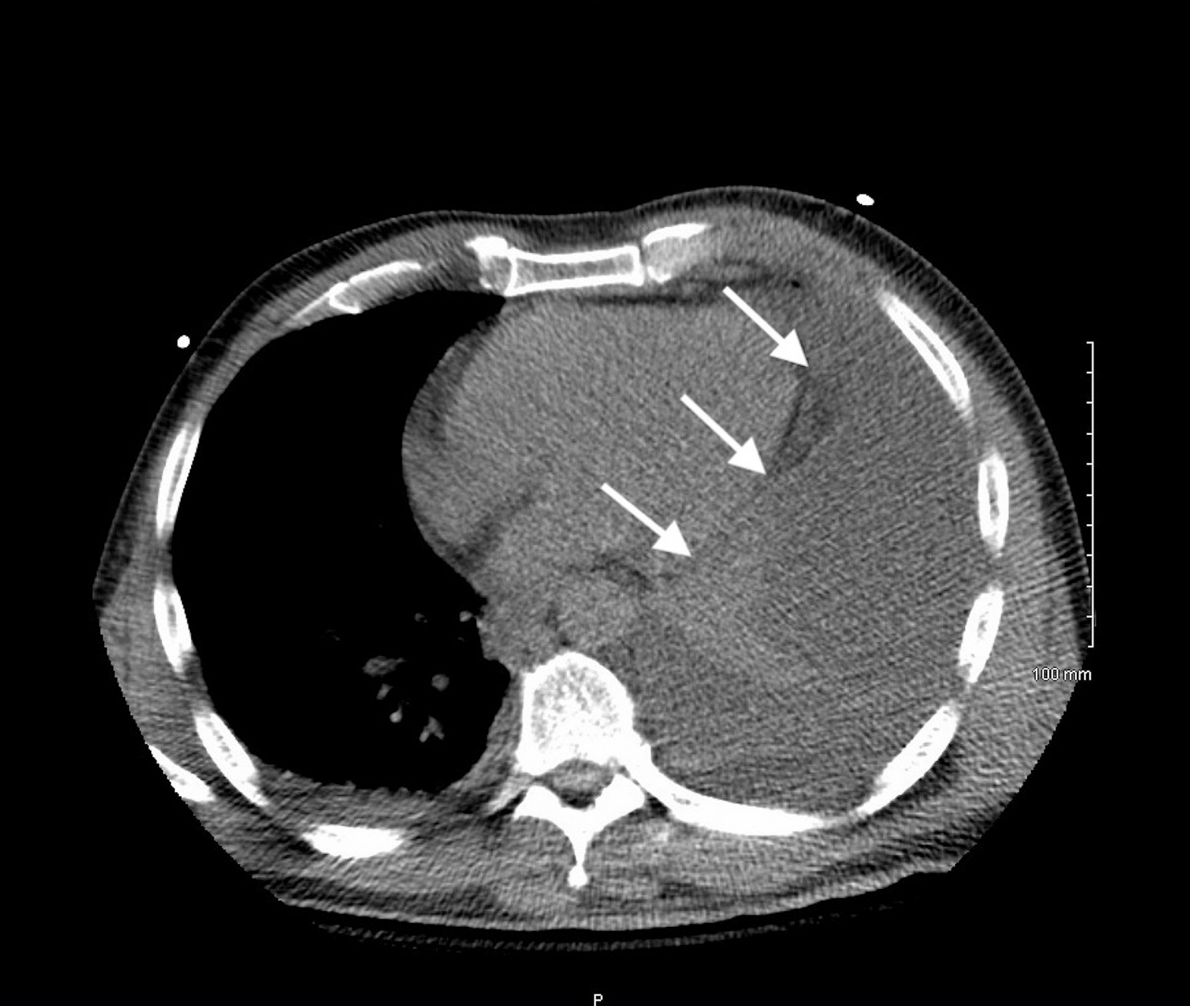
Axial image from non-contrast CT of the chest showing a large, loculated, left-sided pleural effusion bordering the posterolateral margin of the heart (arrows). CT = computed tomography
